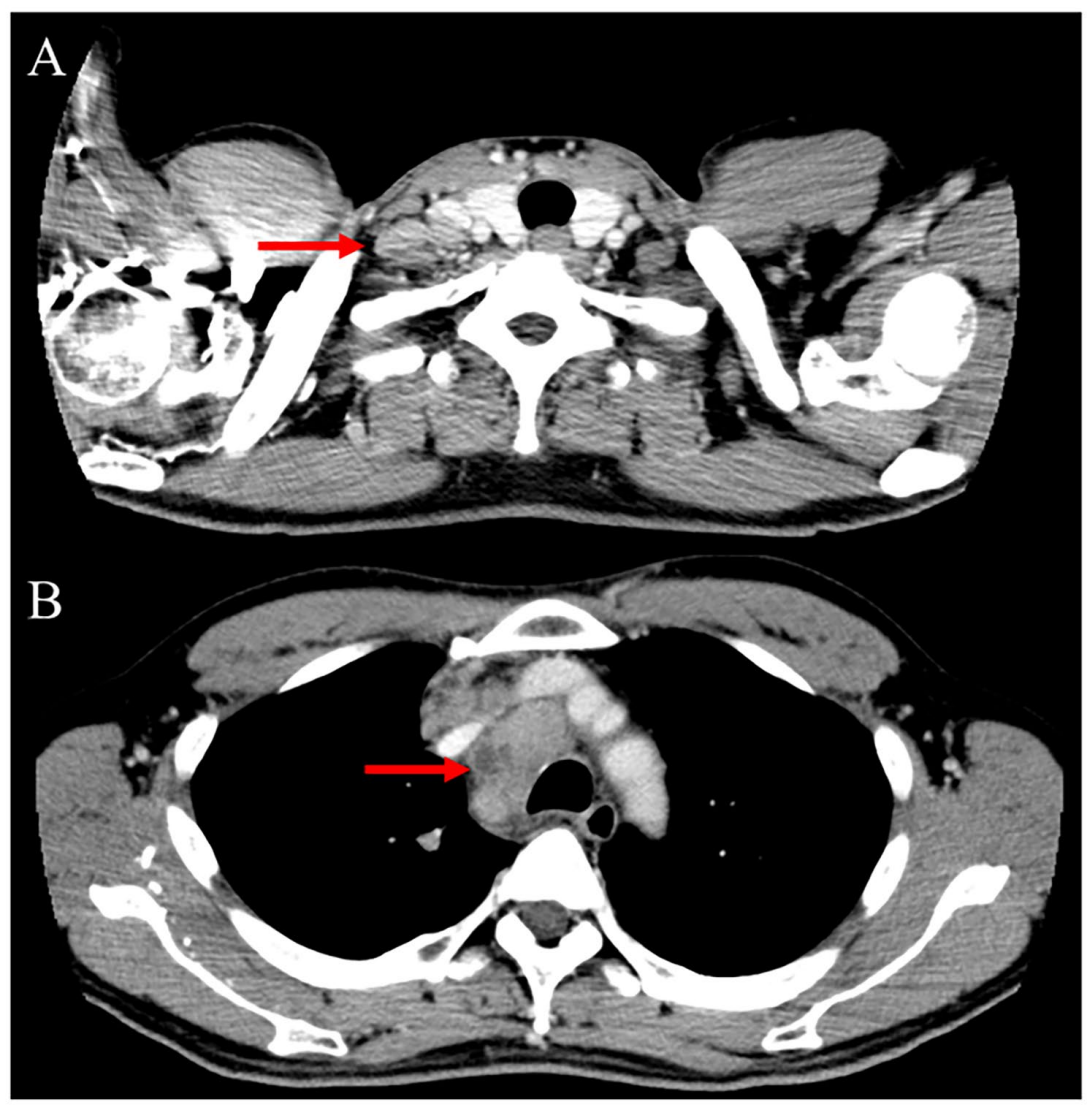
Chest computed tomography. Contrast-enhanced computed tomography showing glioblastoma metastatic lesions in the (A, arrow) right supraclavicular lymph node and (B, arrow) mediastinal lymph node
